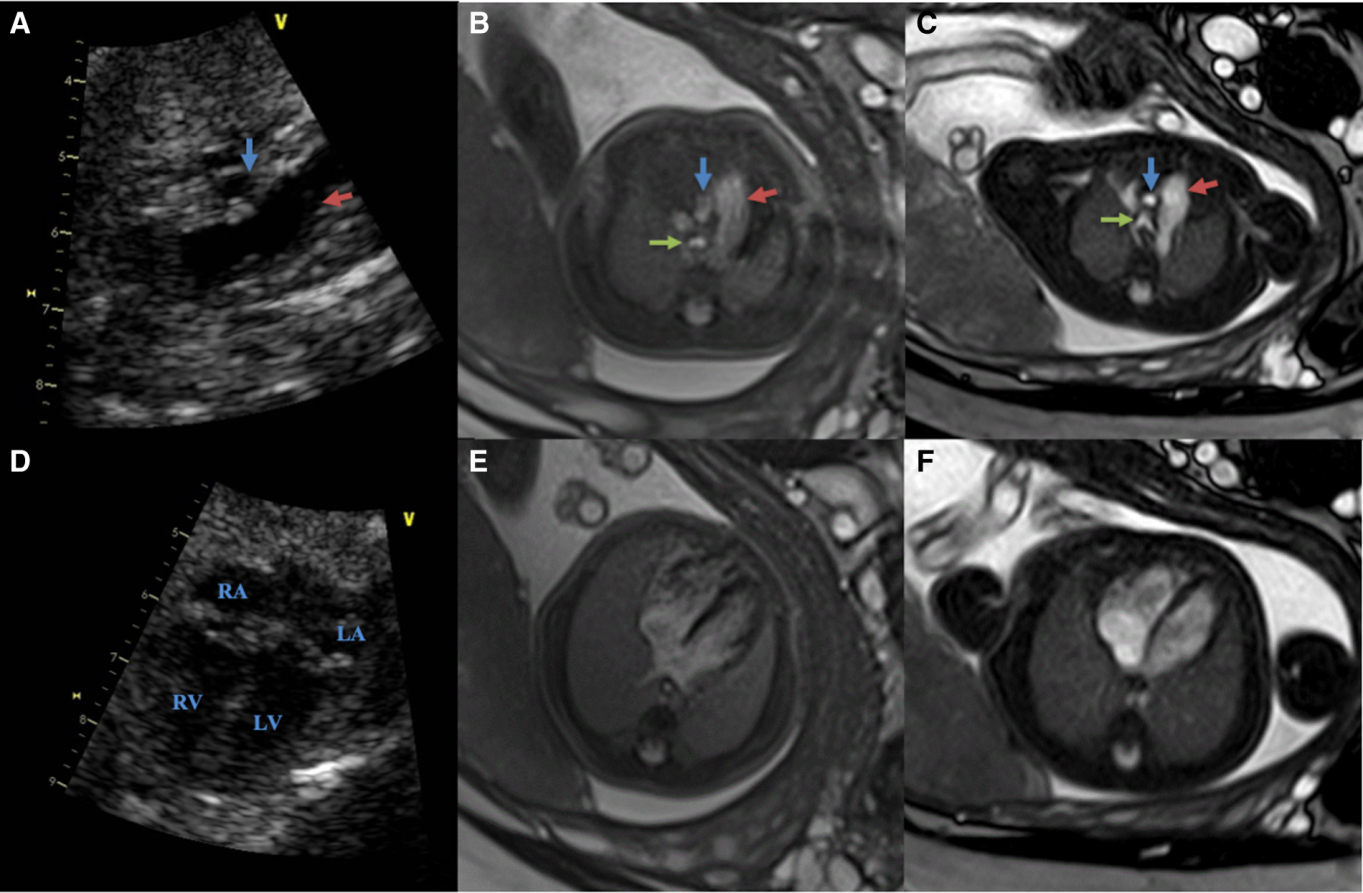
Comparing of the fetal cardiovascular at 26 wk 5 days gestation obtained at 3.0 T, 1.5 T MRI and ultrasound (same fetus) (A–C): ultrasound (A), 3.0 T (B) and 1.5 T(C) in the great vessels slice (red arrows show pulmonary artery; blue arrows show aorta; green arrows show the trachea) (D–F): ultrasound (D), 3.0 T (E) and 1.5 T(F) in the four-chamber slice both 1.5 T and 3.0 T scans were performed on the same day. The images from 1.5 T show superior tissue contrast and conspicuity to that of 3.0 T.
